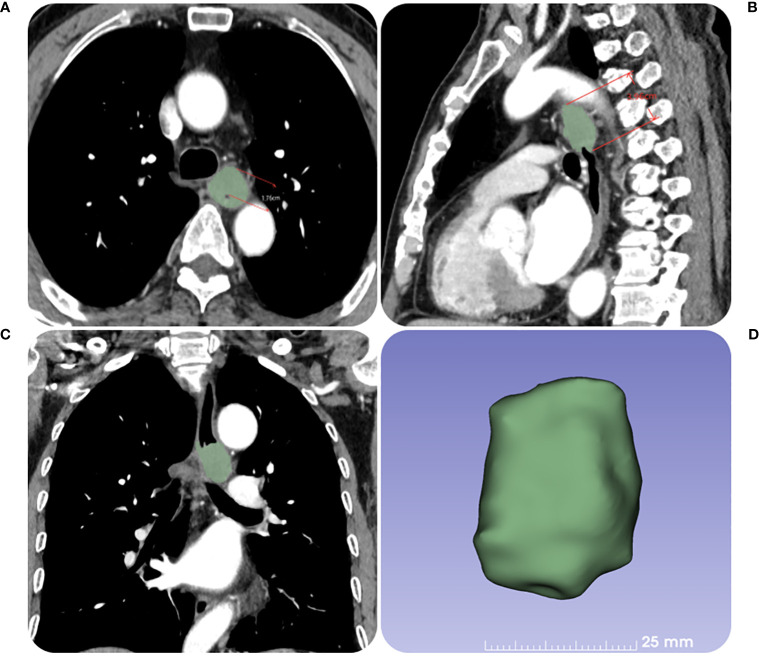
The contrast-enhanced CT images of a 67-year-old female patient with ESCC before RT. (A) The tumor maximal wall thickness is measured in the transverse axial position. (B, C) The tumor length is measured in the sagittal position with reference to the coronal position. (D) The tumor volume is obtained by outlining the tumor layer by layer.
